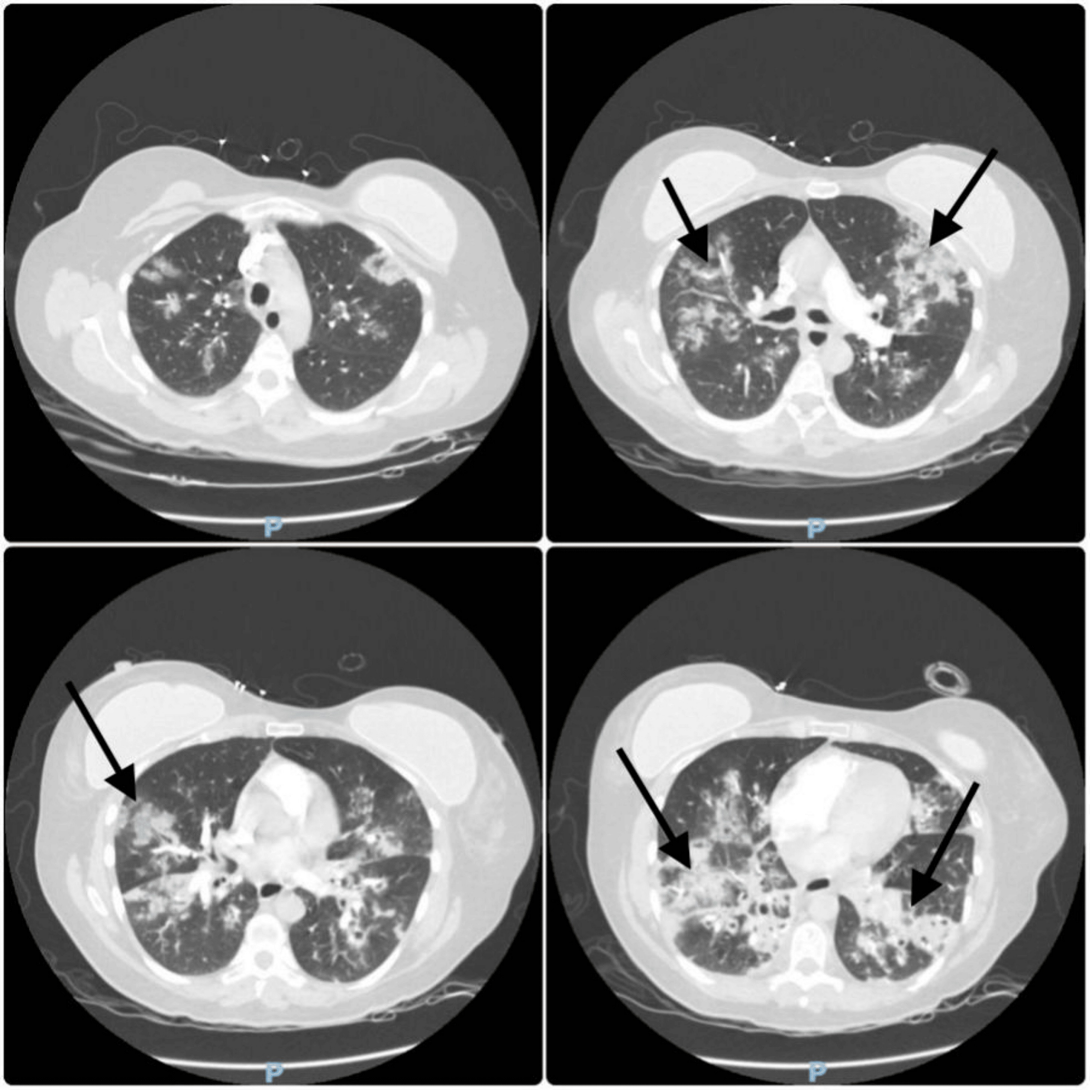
CT chest on admission CT: computed tomography
